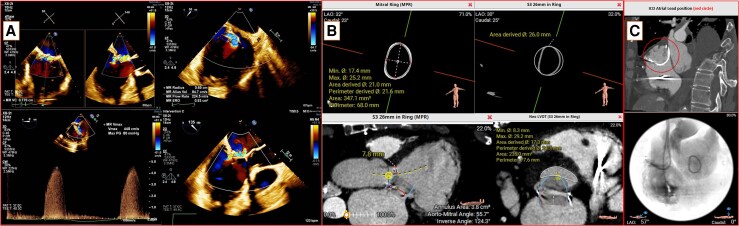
(*A*) Echocardiography assessment showed the recurrence of severe mitral regurgitation. (*B*) CT analysis before valve-in-ring transcatheter mitral valve replacement. (*C*) CT evaluation of implantable cardioverter-defibrillator atrial lead position.
